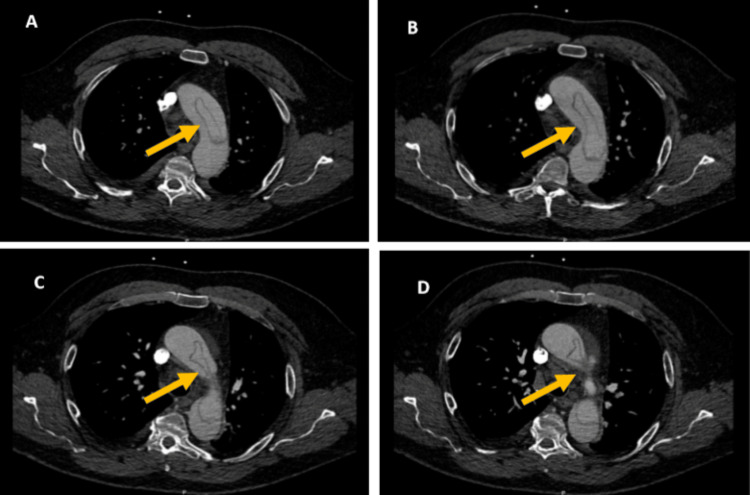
CT angiogram of the aorta showed a Stanford A aortic dissection arising from the aortic sinus and terminating in the infra-renal abdominal aorta. Extension into the brachiocephalic and left subclavian arteries is seen, with the RCA, coeliac, superior and inferior mesenteric arteries arising from the false lumen. The aortic root was dilated to 5.5 cm. RCA: right coronary artery
